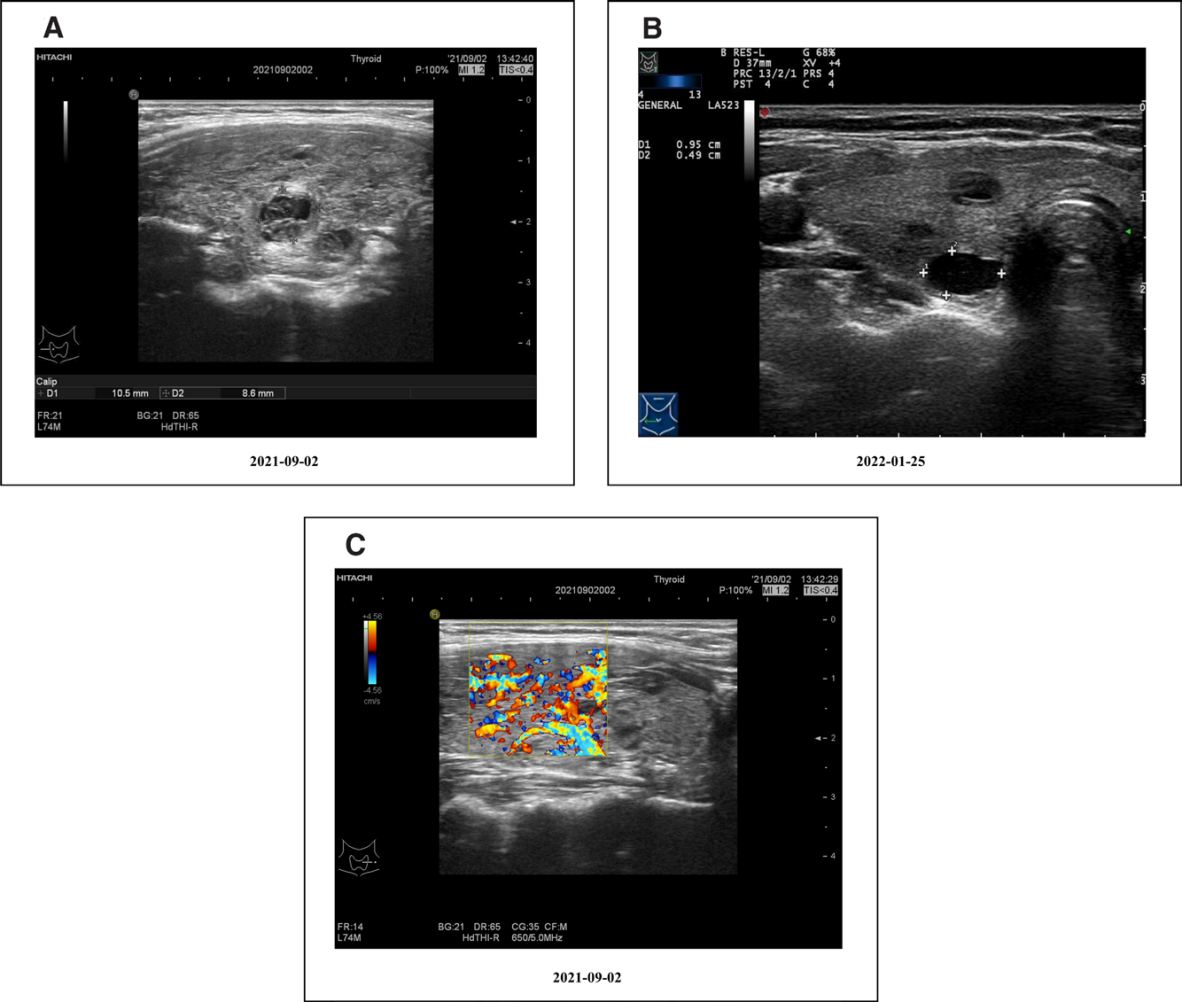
Thyroid ultrasound. (A) Ultrasonic examination of the thyroid showed diffuse thyroid lesions with volume enlargement. (B) Ultrasonic examination of the thyroid showed multiple cystic nodules of the thyroid. (C) Doppler examination of the thyroid showed the “thyroid inferno.”
